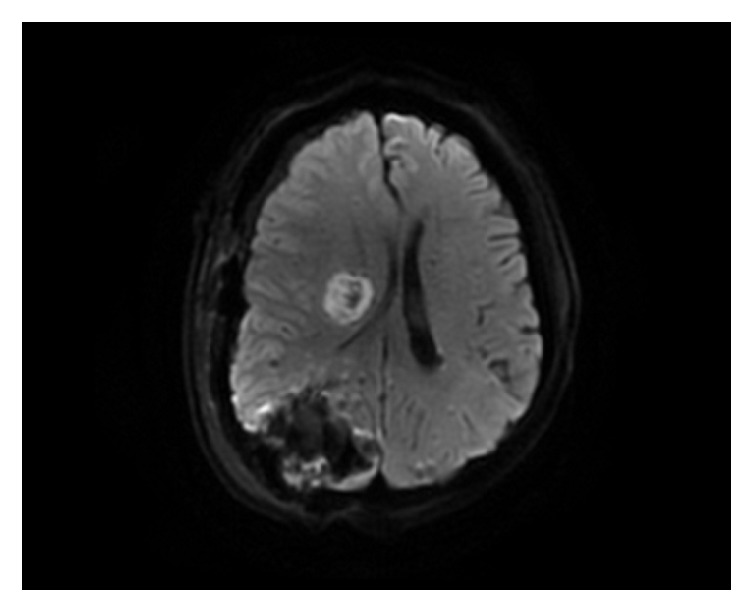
A contrast-enhanced MR imaging of brain performed three days after operation showed irregular mixed signal intensity on DWI with enhancement of its wall in the right occipital lobe and hyperintense lesion with small hypointense areas within in the basal ganglia region.
